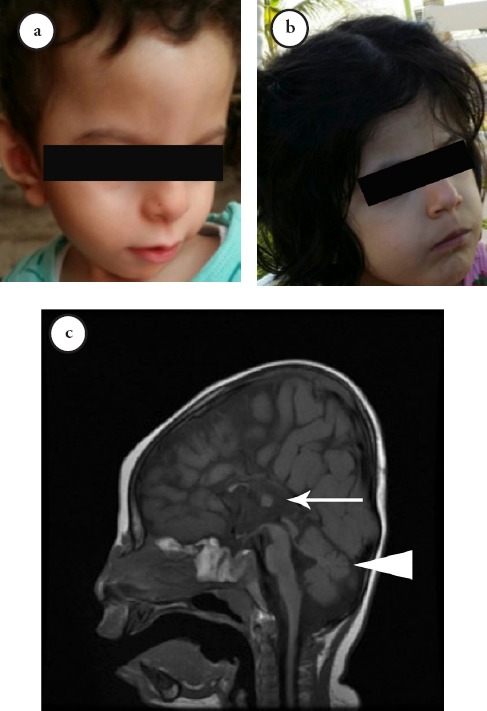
Clinical features of the patients. a, b) The craniofacial features include dysmorphism in the form of wide forehead, hypotelorism, and upturned nose. c) The brain MRI indicates complete agenesis of the corpus callosum (white arrow) with inferior vermis hypoplasia (arrowhead).
